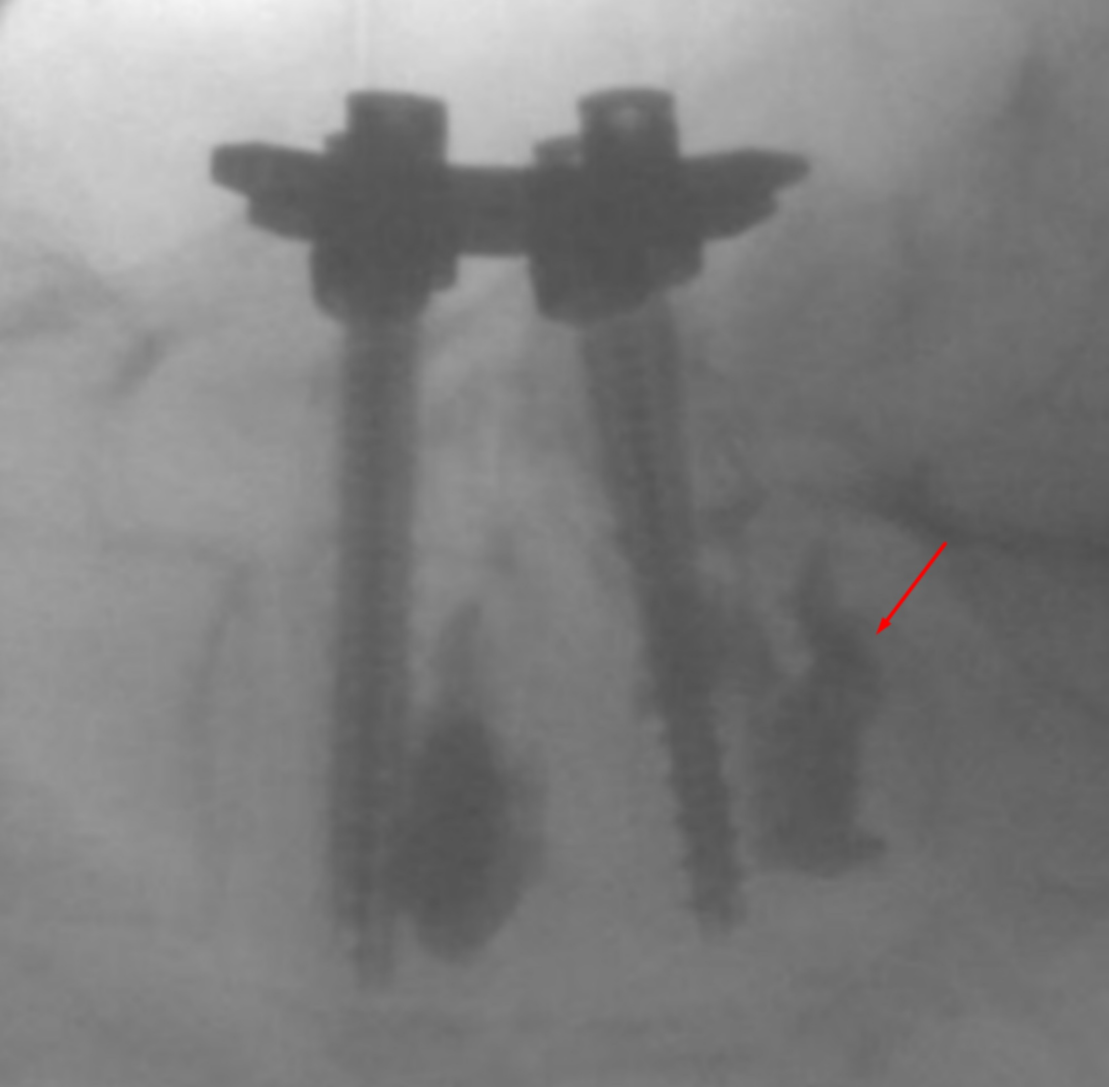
Lateral View - Fixation Final lateral fluoroscopic X-rays demonstrating final fixation of the bone cement. Arrow indicates the bone cement.
